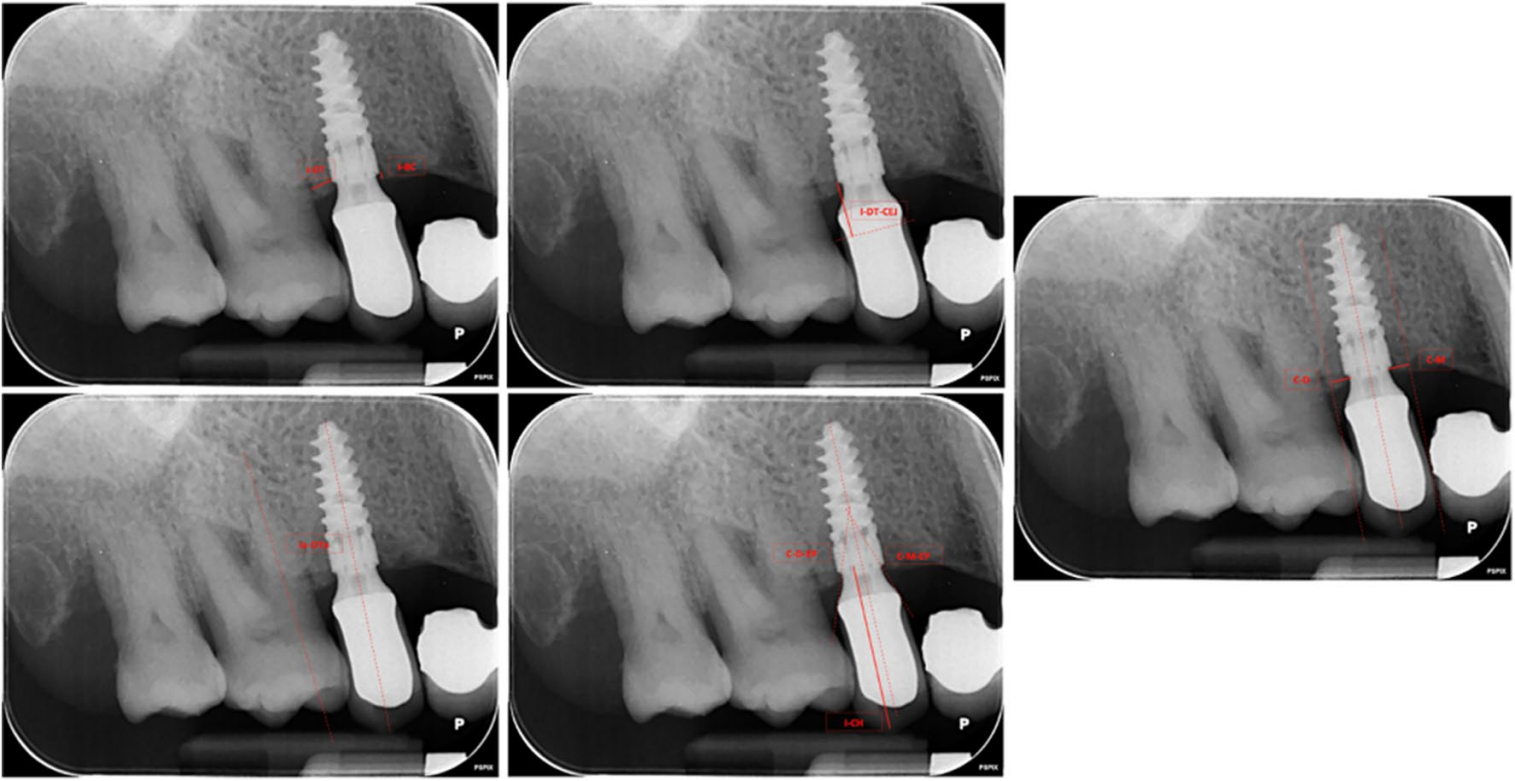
The figure shows, on a periapical radiograph, the parameters that were measured and the respective reference points
